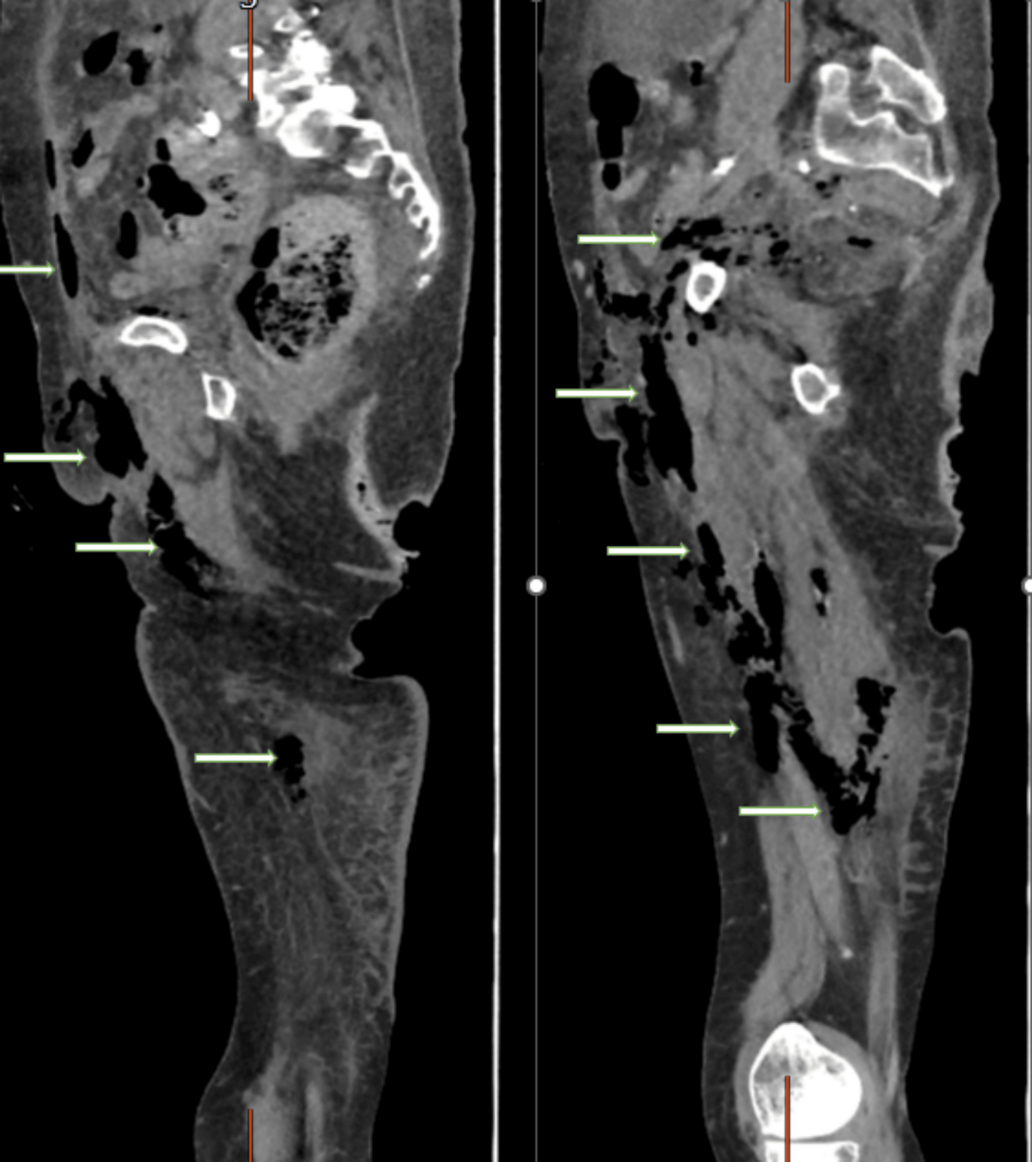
Sagittal plane of a CT scan showing surgical emphysema, indicated by arrows.
